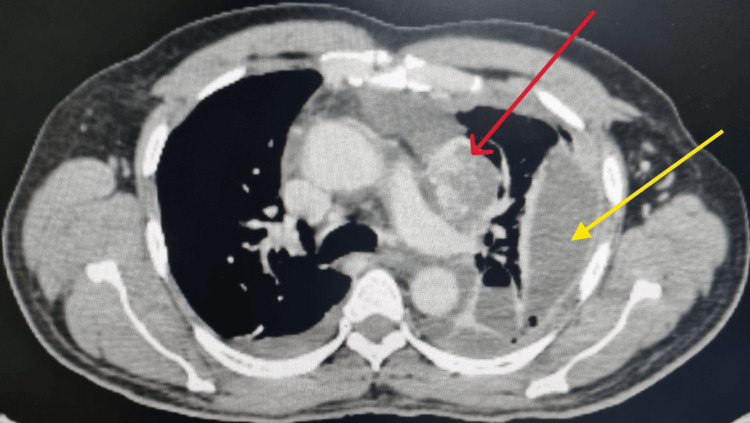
Thoracic computed tomography showing a necrotic mass lesion adjacent to the left main pulmonary artery (red arrow) and pleural effusion (yellow arrow).
